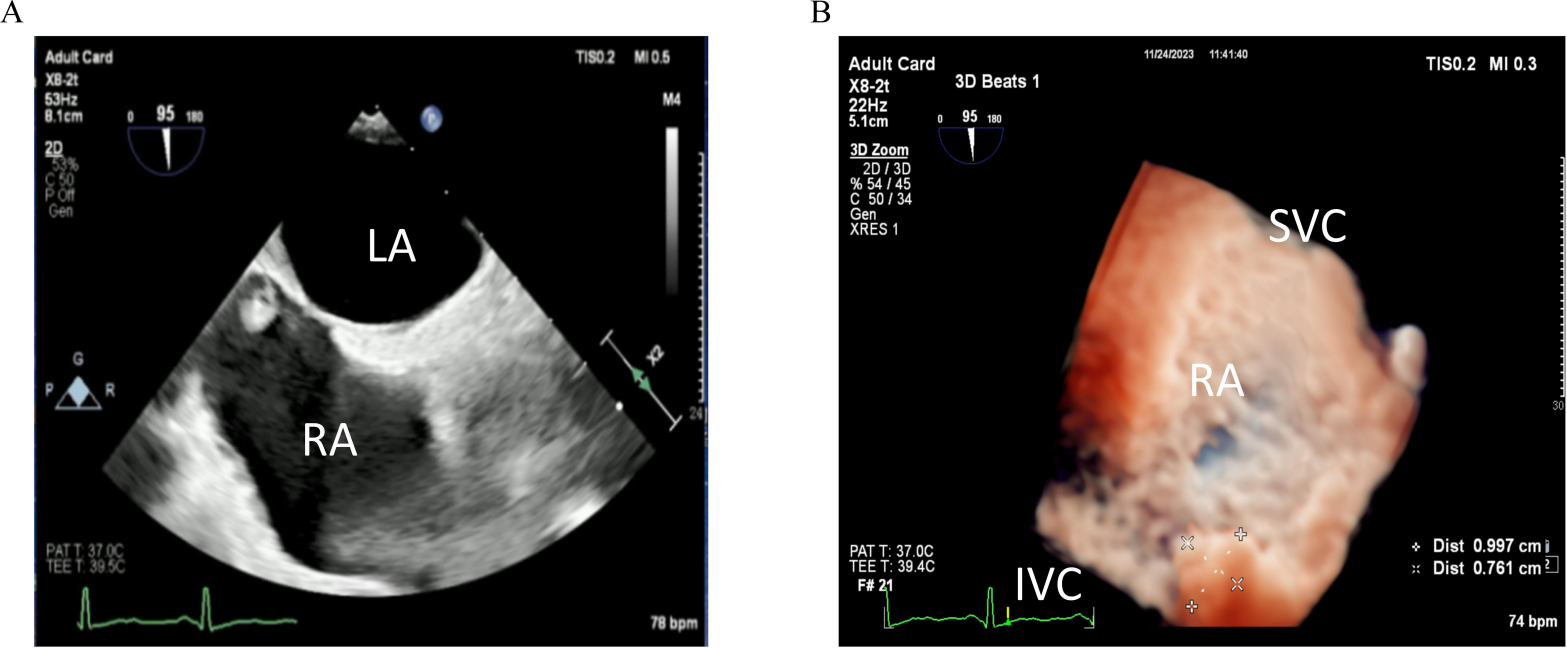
(A) Two-dimensional transesophageal echocardiography image obtained before admission shows a slightly echogenic nodule near the entrance of the inferior vena cava at the top of the right atrium (arrow). (B) Three-dimensional transesophageal echocardiography image obtained before admission shows a nodule near the entrance of the inferior vena cava at the top of the right atrium (arrow).
